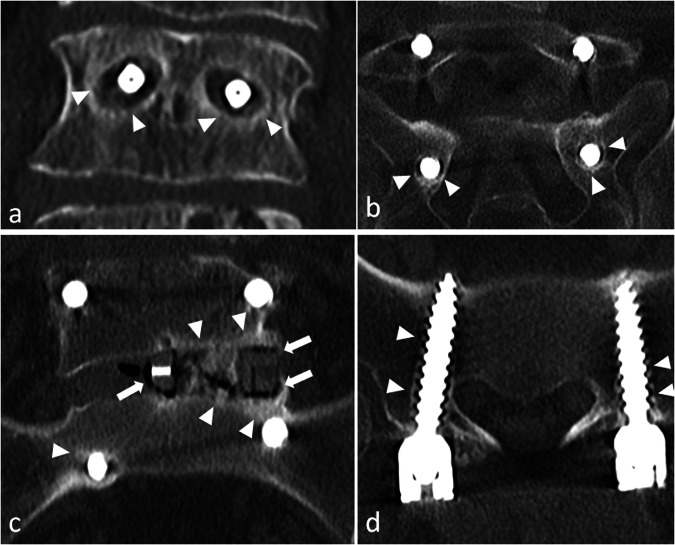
Typical aspects of mechanical pseudarthrosis in this patient who benefited from a posterior lumbar intervertebral fusion with an interbody spacer. Wide osteolysis with sclerotic margins realise the typical aspect of owl eyes on coronal CT images (arrowheads) (a). An identical aspect is seen all along the inferior screws (arrowheads) on coronal (b) and axial (d). Gas around and inside the interbody spacer (arrows) is the vacuum phenomenon secondary to micromobility. Osteosclerosis of the adjacent vertebral endplates (arrows c) and around the tip of one screw is also present because of this micromobility (d)
